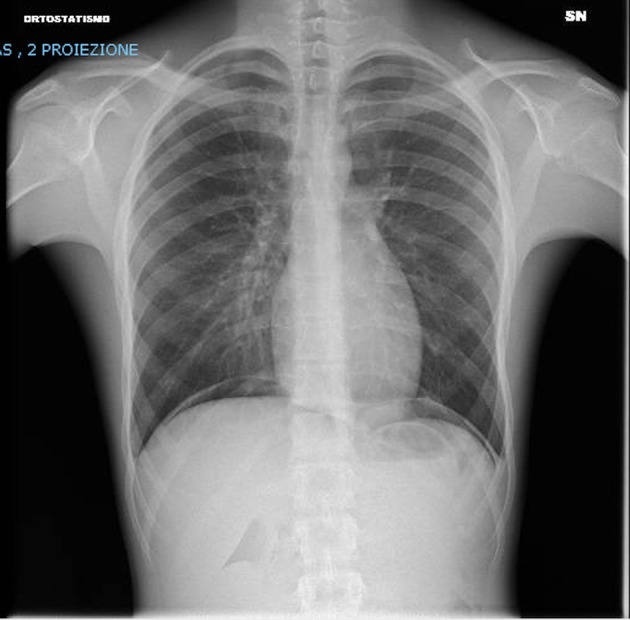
Chest x-ray after 48 h showing both lungs clear of significant parenchymal opacities and no signs of pleural effusions.
